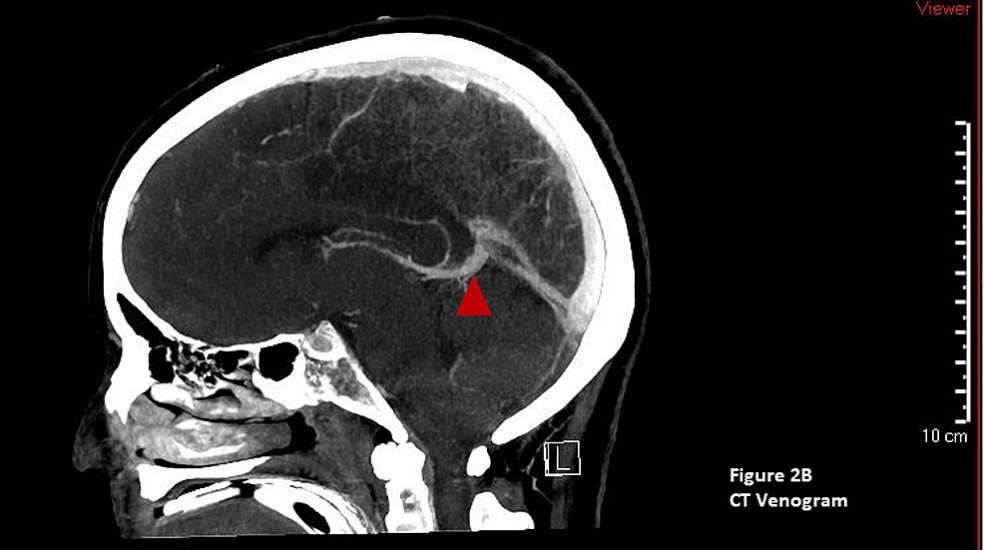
CT venogram of the head. The red triangle shows normal venous blood flow.
